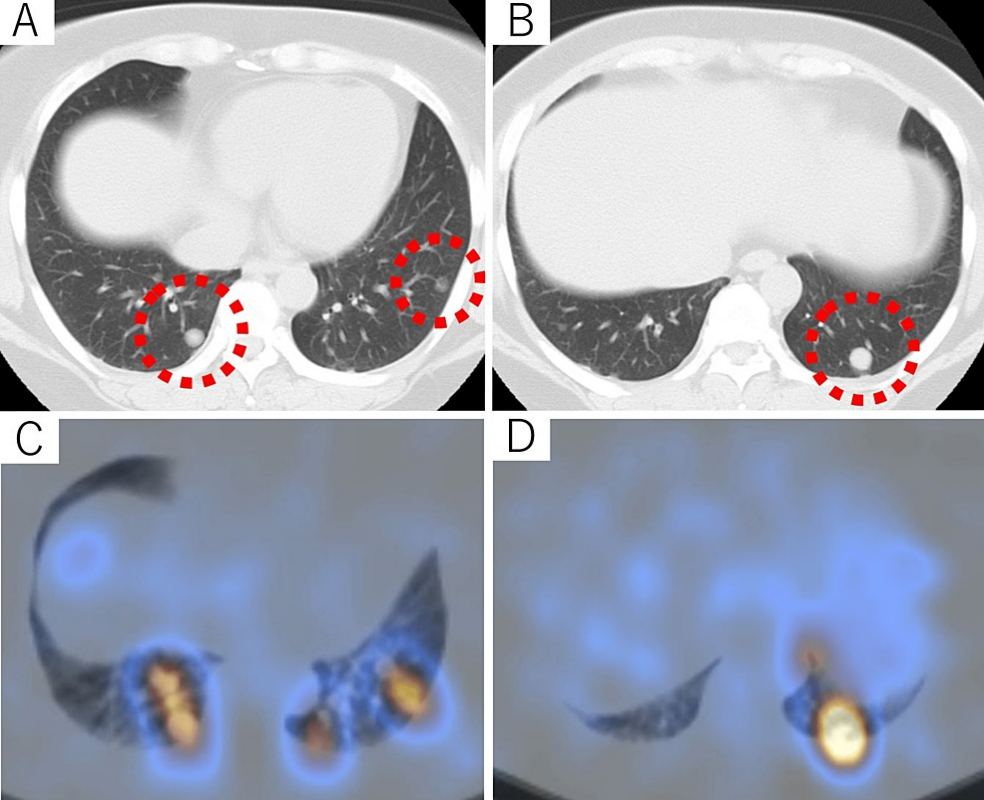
Representative images of the multiple pulmonary metastases (A, B) A chest CT scan performed at the time of the initial diagnosis of pulmonary metastases. (C, D) Scintigraphy with SPECT/CT performed five days after 131I administration. CT: computed tomography; SPECT: single photon emission computed tomography; 131I: iodine-131.
